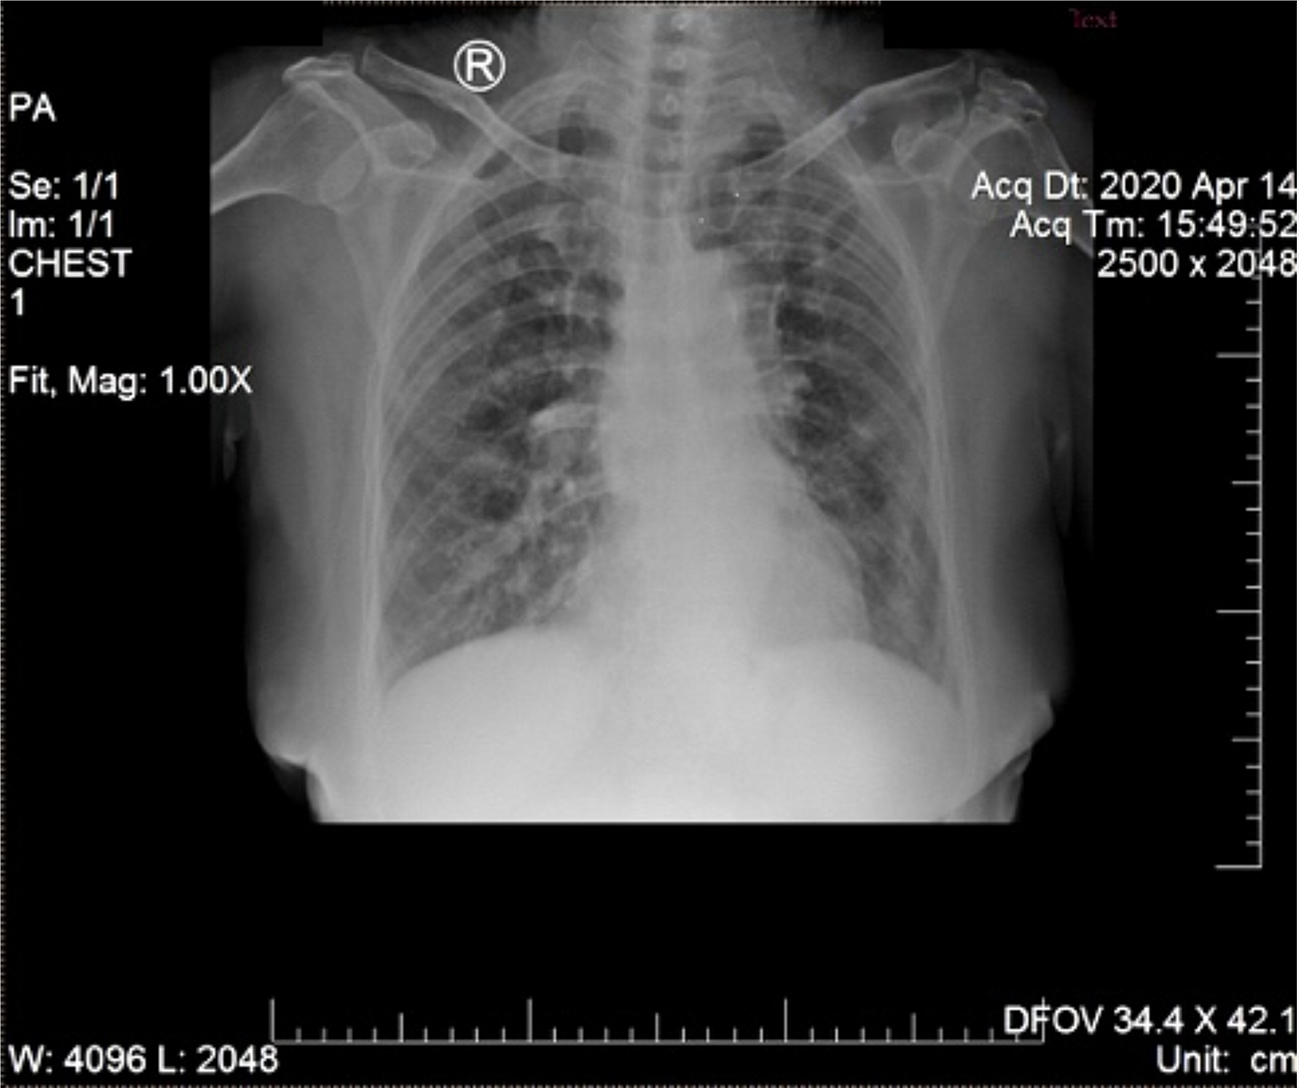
Chest X-ray on day of hospitalization. Pericardial infiltrates suggest bronchopneumonia.
